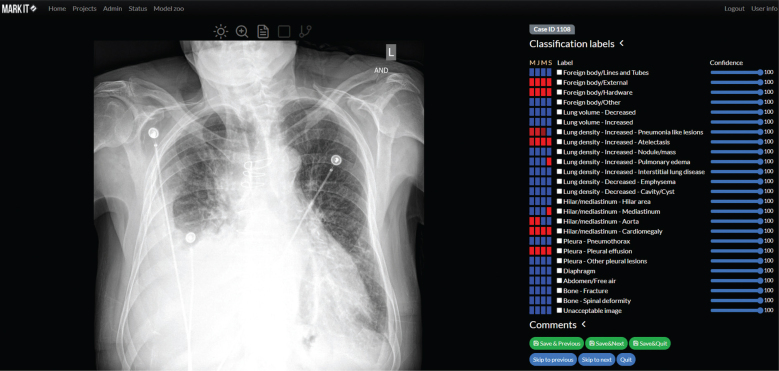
Main module for image annotation combining basic DICOM viewer features (i.e. change brightness or contrast, zooming, etc.), displaying radiological reports and annotation tools (above the X-ray image). Annotators can determine their confidence with regard to each label (blue bars on the right) and preview annotators by other team members (blue and red rectangles).
